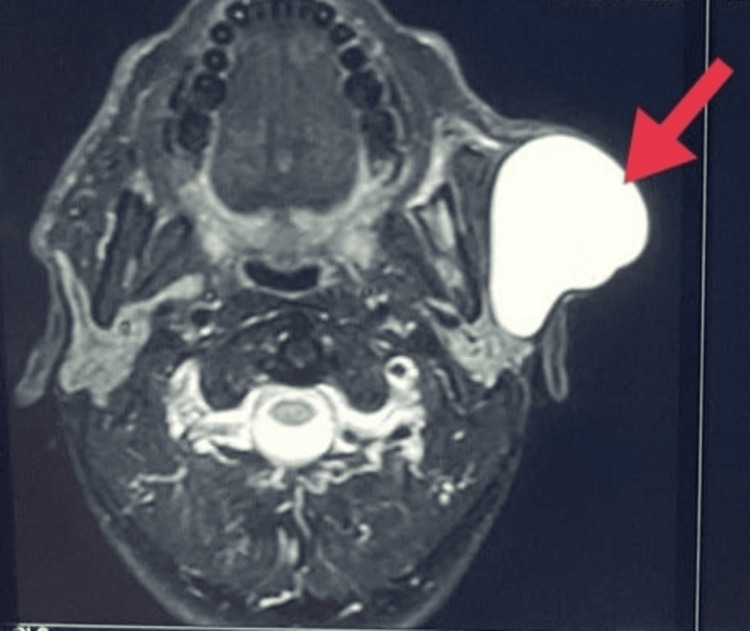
T2-weighted MRI image (axial section) showing a well-defined hyperintense lesion in the subcutaneous plane abutting the parotid gland
